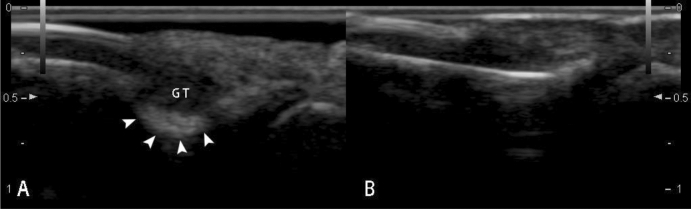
19-year-old female with painful nodule below the right index finger. Sonography shows a hypoechoic nodule (5.1 mm in length and 2.6 mm thickness) in the subungual region, four arrow heads point to erosion of distal phalanx (A). Sonography shows the normal cortex of distal phalanx in the contralateral index finger (B).
